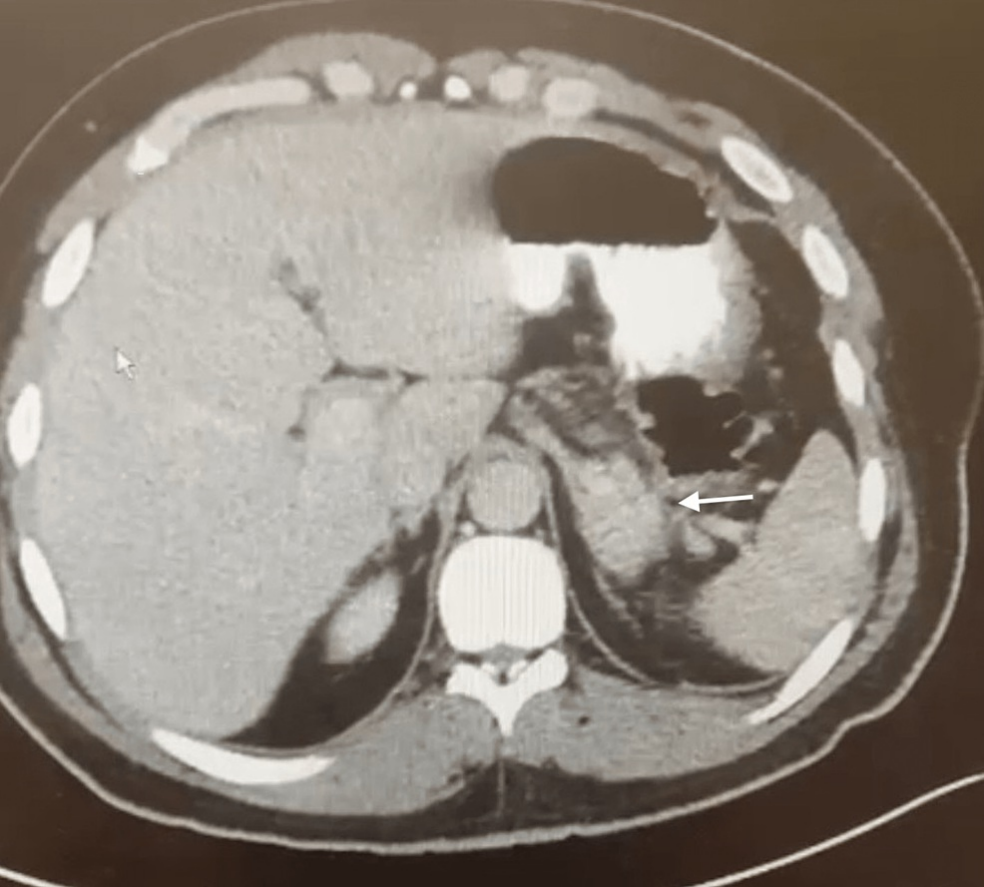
CT of the abdomen with IV contrast showing faint peripancreatic fat stranding adjacent to the pancreatic tail
